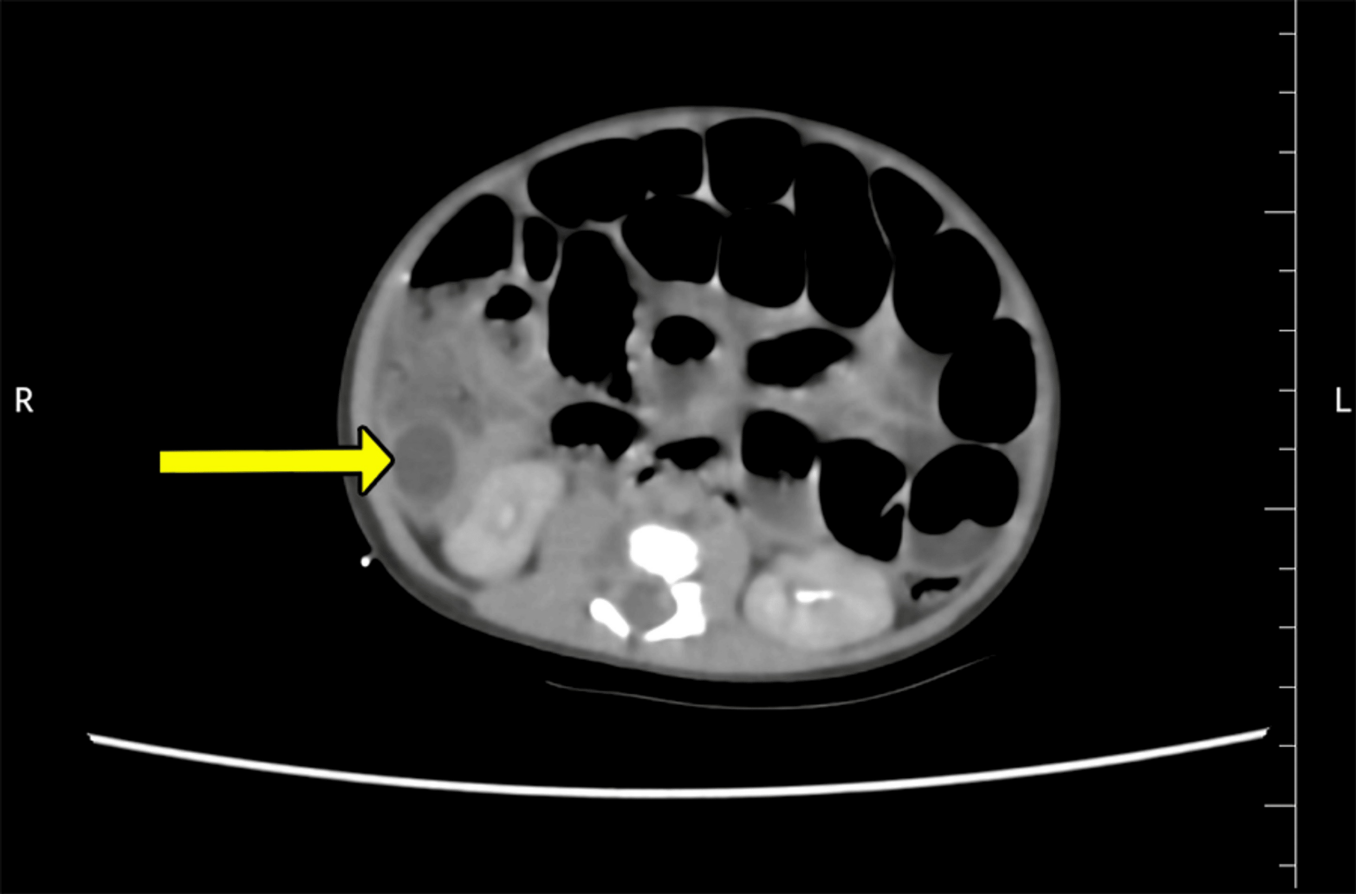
Contrast-enhanced computed tomography (CECT) of the abdomen and pelvis Demonstrating a well-defined cystic lesion in the terminal ileum, abutting the cecum and causing obstruction (yellow arrow)
